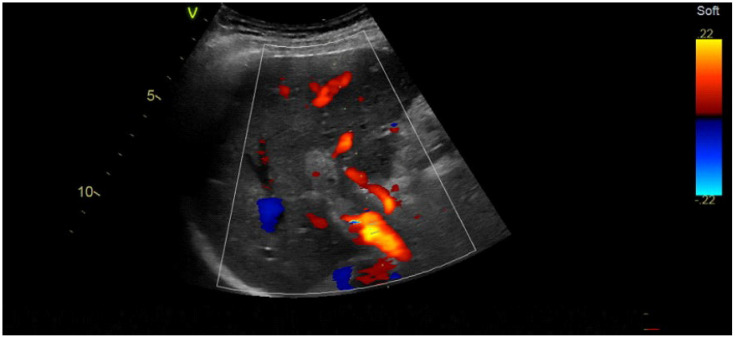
Doppler ultrasound imaging of the portal vein thrombosis.
